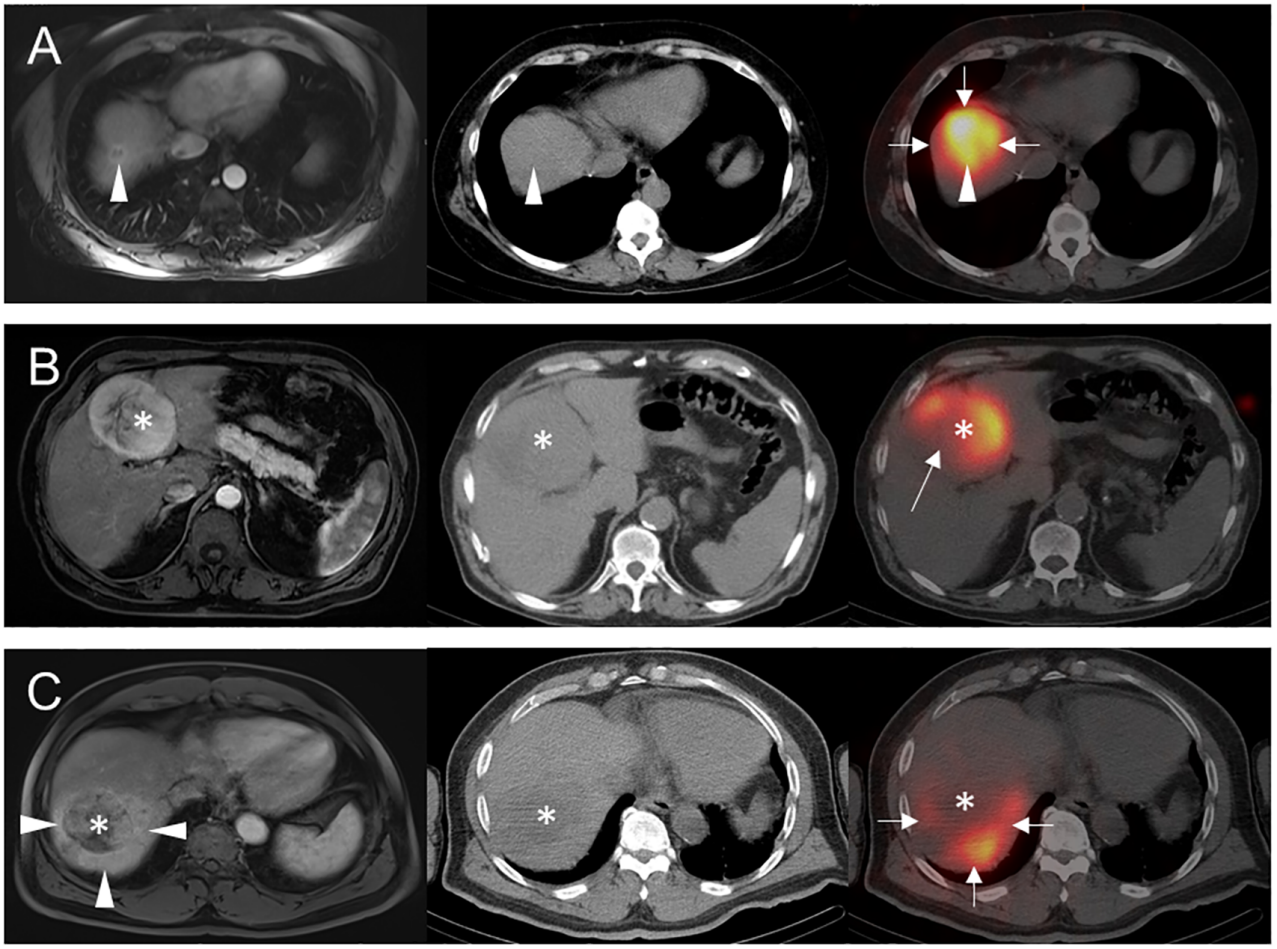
Examples of arterial perfusion categorizations. Pre-treatment axial arterial phase post-contrast T1-weighted MR images (left column) prior to Y-90 TARE and post-treatment axial noncontrast CT (middle column) and fused Y-90 SPECT/CT images (right column) are shown for 3 separate patients. (A) 56-year-old female with segment 8 intrahepatic cholangiocarcinoma (arrowheads) underwent Y-90 TARE, with post-treatment Y-90 SPECT/CT showing uniform activity throughout segment 8 (arrows), including the target lesion (arrowhead). This tumor was classified as hyperperfused, based on its activity similar to background liver parenchyma. (B) 85-year-old male with segment 4B HCC (asterisks) underwent Y-90 TARE with post-treatment Y-90 SPECT/CT showing an area of near-absent Y-90 activity (arrow) in the right posterolateral aspect of the tumor, presumably due to interval necrosis versus partial arterial supply by an unmapped hepatic arterial branch. Given these findings, the tumor was classified as hypoperfused. (C) 80-year-old male with segment 7 HCC (asterisks) underwent Y-90 TARE with post-treatment Y-90 SPECT/CT showing tumor activity substantially less than background (arrows), potentially related to peritumoral shunting as seen on prior MRI (arrowheads). Based on these findings, the tumor was classified as hypoperfused.
